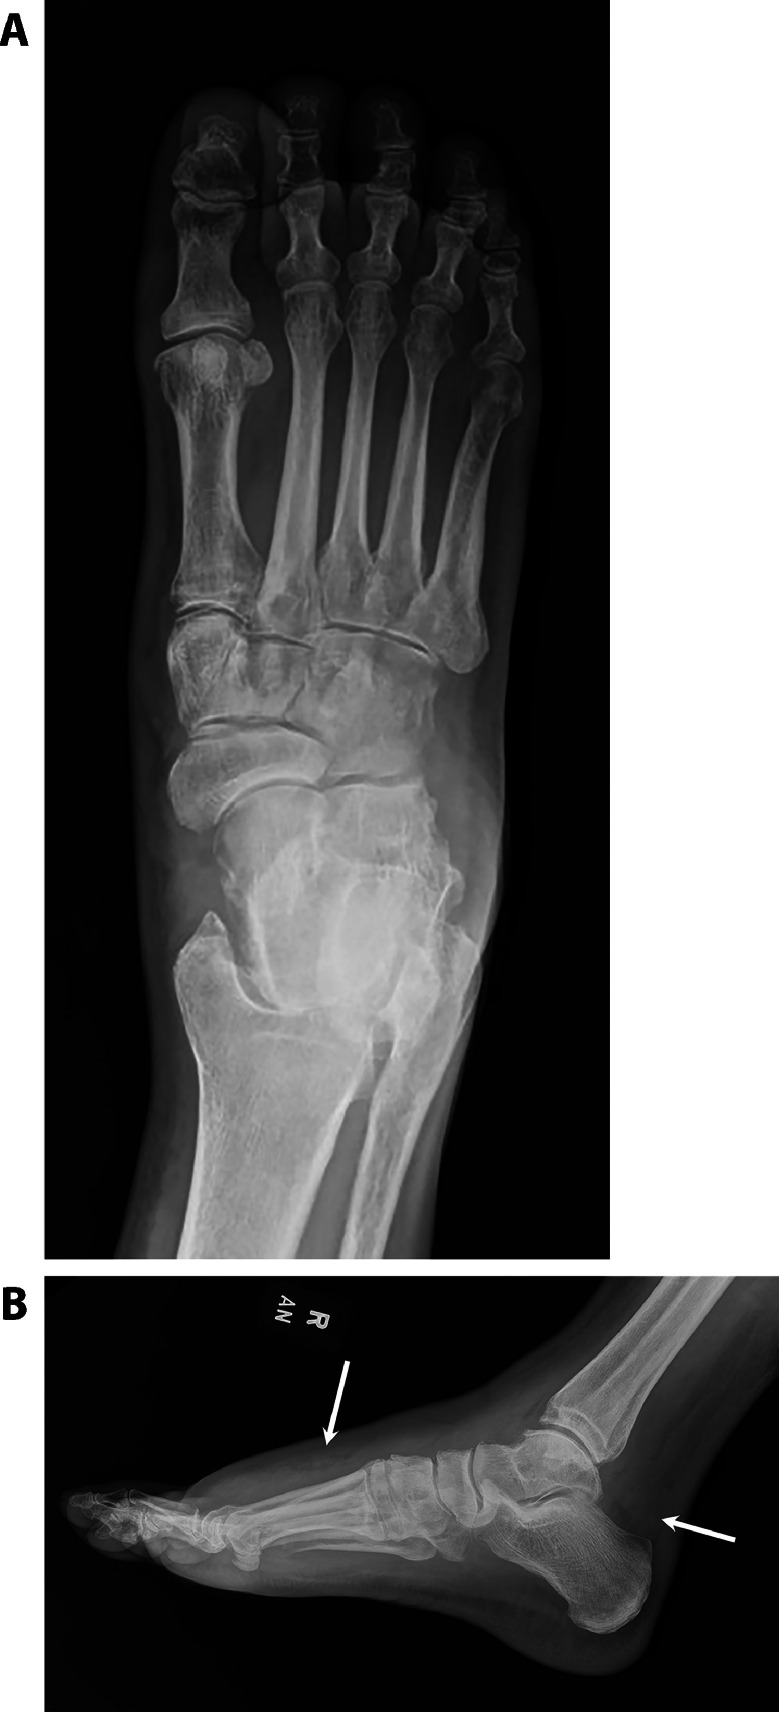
(A) AP radiograph of the right foot demonstrates no acute osseous abnormality. No definite erosions are seen. (B) Lateral radiograph of the right foot demonstrates areas of soft tissue swelling at the dorsal foot and posterior ankle (white arrows).
